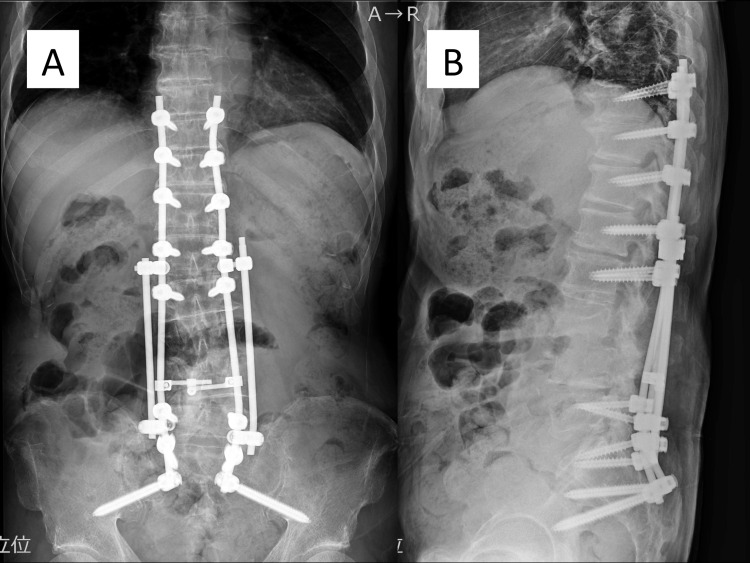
Postoperative X-ray of anterior debridement and autogenous bone grafting to L3/4. (A) Anteroposterior X-ray image. (B) Lateral X-ray image.
